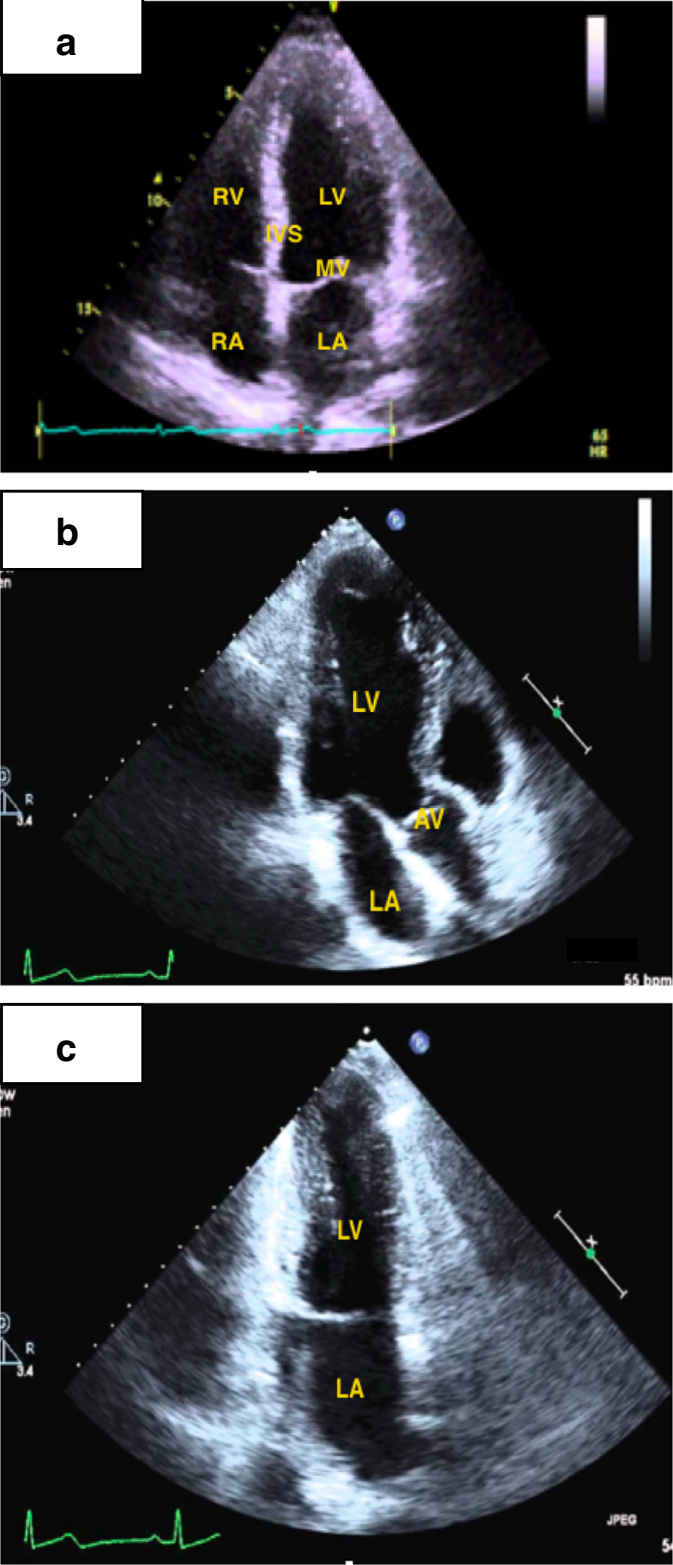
Apical 4, 3 and 2 chamber views. a Aplical 4 chamber view. b Aplical 3 chamber view. c Aplical 2 chamber view
